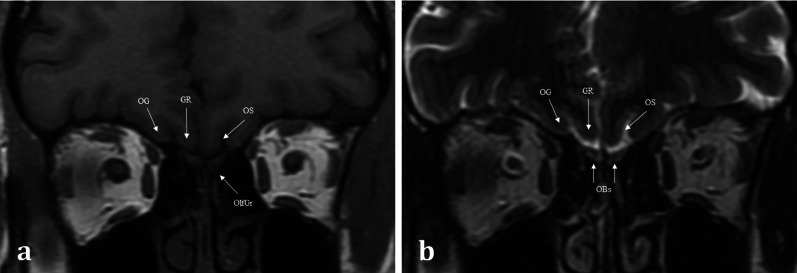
Normal Anatomy. Coronal T1WI (a) and T2WI (b) MRI images of the normal location and appearances of the olfactory bulbs (OBs). These lie within the olfactory grooves (OlfGr) of the anterior cranial fossa. Notice how the olfactory bulbs are surrounded by T2 hyperintense CSF. The olfactory tracts (not shown) are a posterior continuation of the olfactory bulbs and run within the olfactory sulci (OS). The olfactory sulcus is lateral to the gyrus rectus (GR) and medial to the orbital gyrus (OG)
